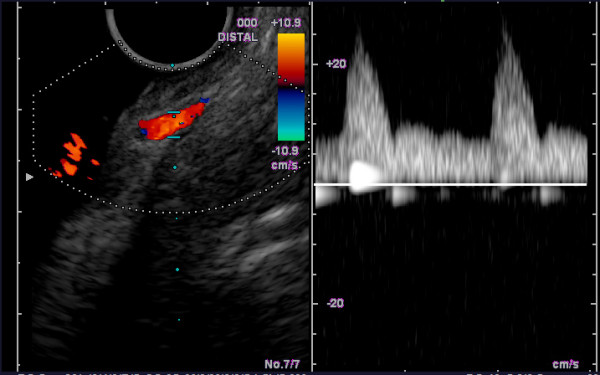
Endoscopic colour duplex sonography showing arterial flow on the apparently gastric varices.
